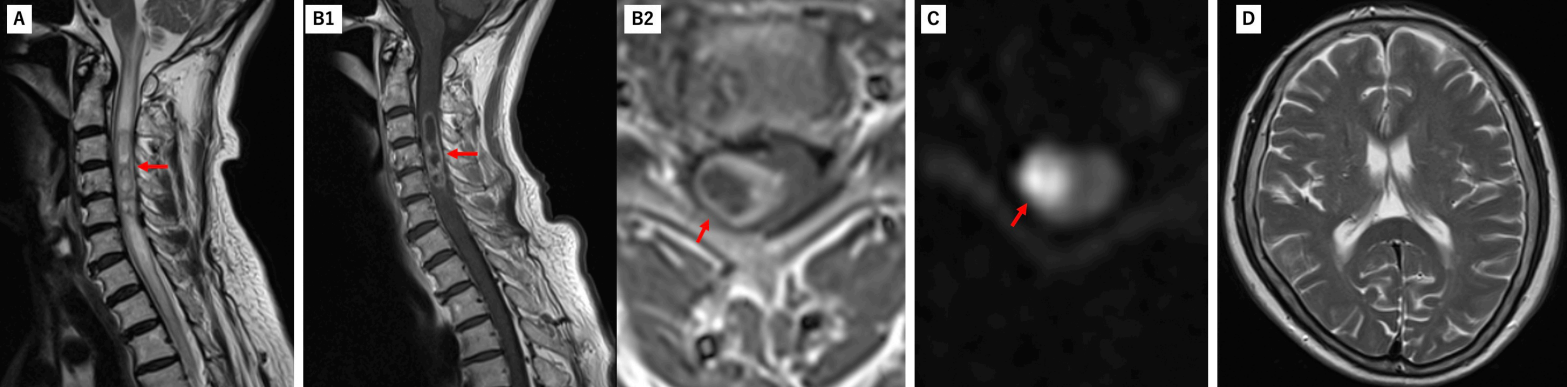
On the second day of hospitalization, a follow-up MRI showed progressive lesion expansion (red arrow) with worsening edema and ring-like enhancement on contrast-enhanced MRI, and high signal intensity on diffusion-weighted imaging in the same region. Brain MRI showed no changes compared to admission, with no hydrocephalus or findings suggestive of brain abscess or ventriculitis. (A: T2WI Sagittal; B1, 2: gadolinium-enhanced T1WI Sagittal, Axial; C: DWI Axial; D: T2WI Axial). MRI: magnetic resonance imaging; T2WI: T2 weighted image.
